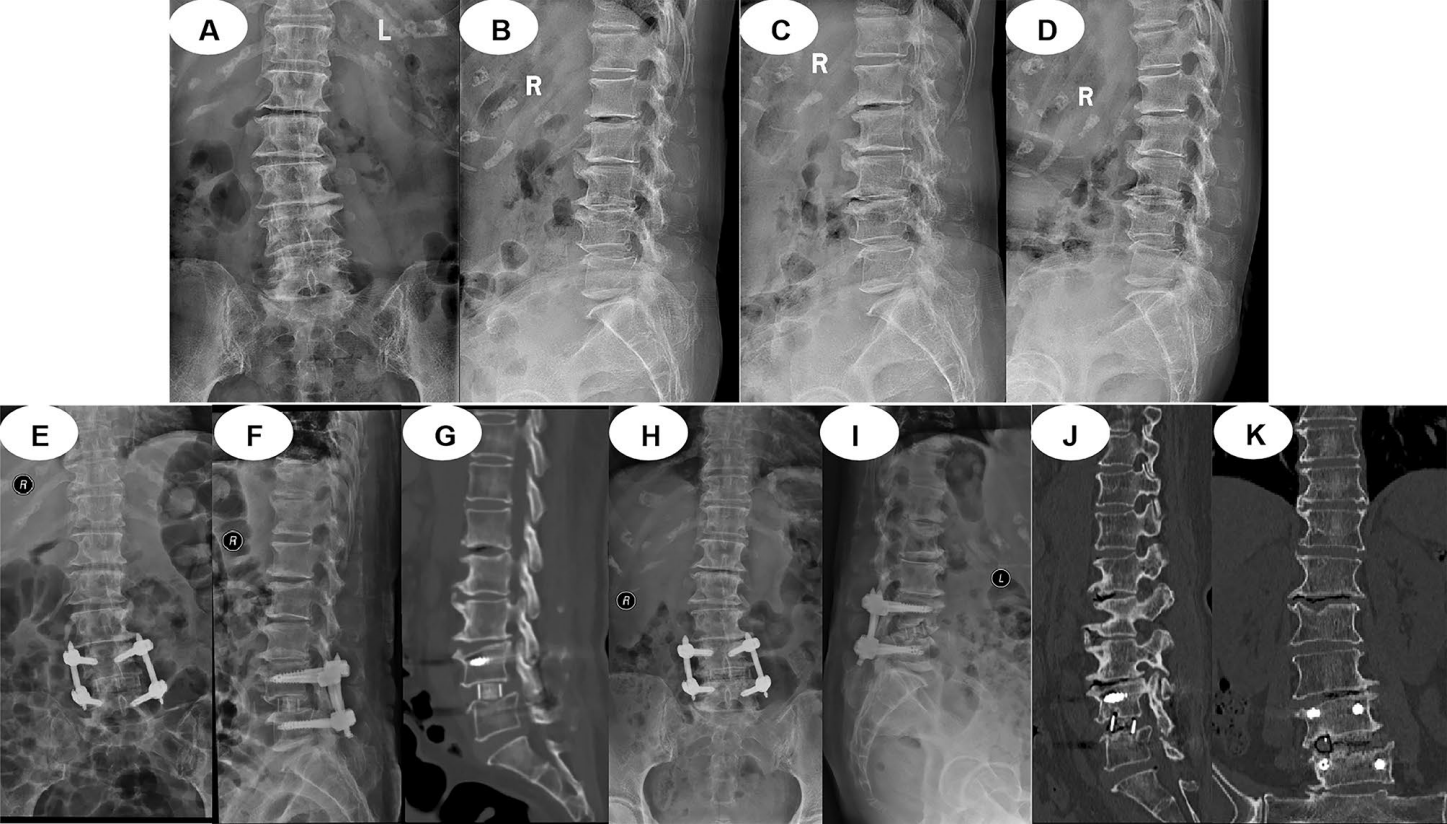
A 74-year-old woman had cage subsidence after OLIF. Preoperative lumbar spine anteroposterior and lateral radiographs (A, B), lumbar flexion–extension stress lateral radiographs (C, D). X-ray radiographs (E, F) and CT (G) at 1 month postoperatively show that the cage was in a good position. X-ray radiographs (H, I) and CT (J, K) at 3 months postoperatively showed cage subsidence.
